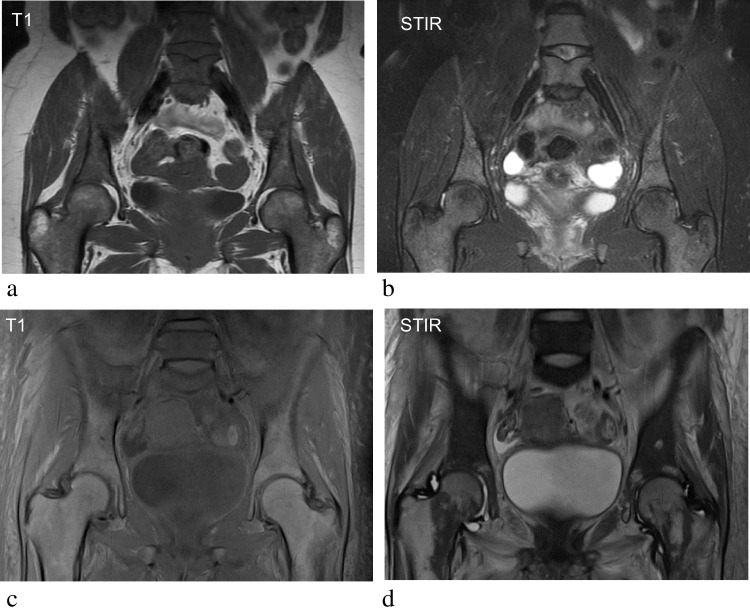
Bone marrow reconversion in a 56-year-old female with sickle cell disease. Sagittal T1-weighted image (a) demonstrates diffuse hypointense marrow signal in the pelvis and proximal femora with corresponding mild hyperintensity on T2 fat-saturated sequences (b). Typical H-shaped vertebrae secondary to sickle cell disease are also demonstrated. In contrast to SABM, there is normal T1-weighted signal of the subcutaneous tissues (a) and expected suppression of fat on the STIR sequences (b). Therefore, bone marrow reconversion is an important differential diagnosis of diffuse bone marrow signal changes, but without the characteristic soft tissue signal abnormalities seen in SABM. Sagittal T1-weighted (c) and T2-weighted fat-saturated sequences (d) in SABM from Fig. 4 for comparison, demonstrating characteristic inverted signal changes of the bone marrow with diffuse signal changes in the subcutaneous tissues
